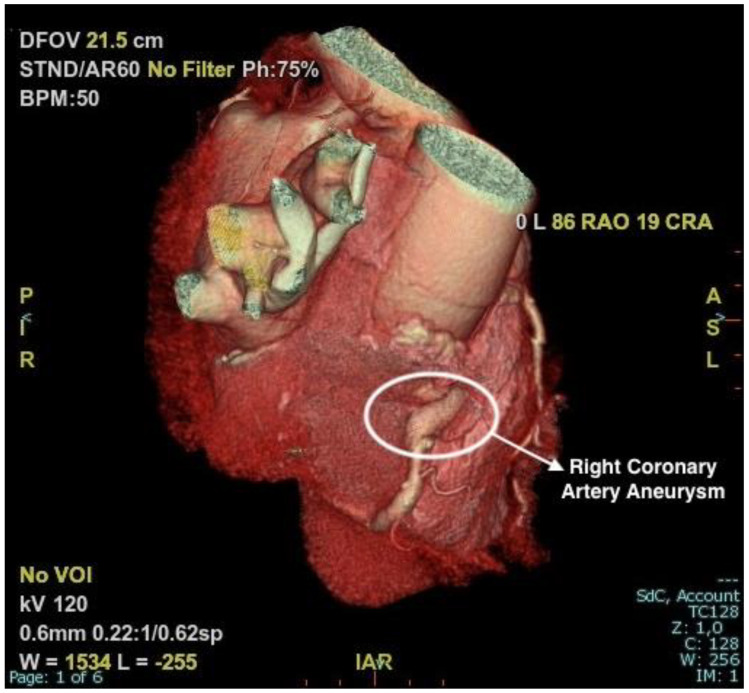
Three-dimensional reconstruction of CT for viewing the right coronary artery, highlighting the aneurysmal formation in the middle segment and its relationship with surrounding anatomical structures.
